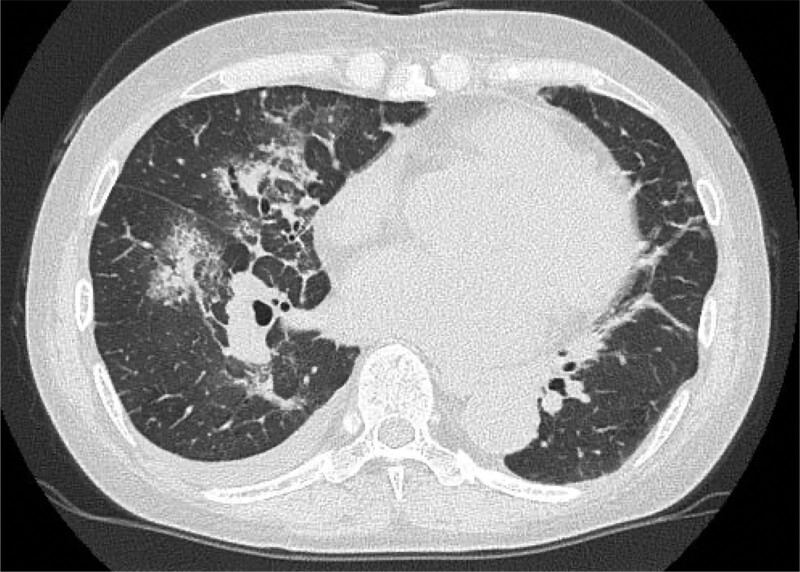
Thoracic CT imaging. Thoracic CT showing diffuse lung consolidation in the right lung. CT = computed tomography.
